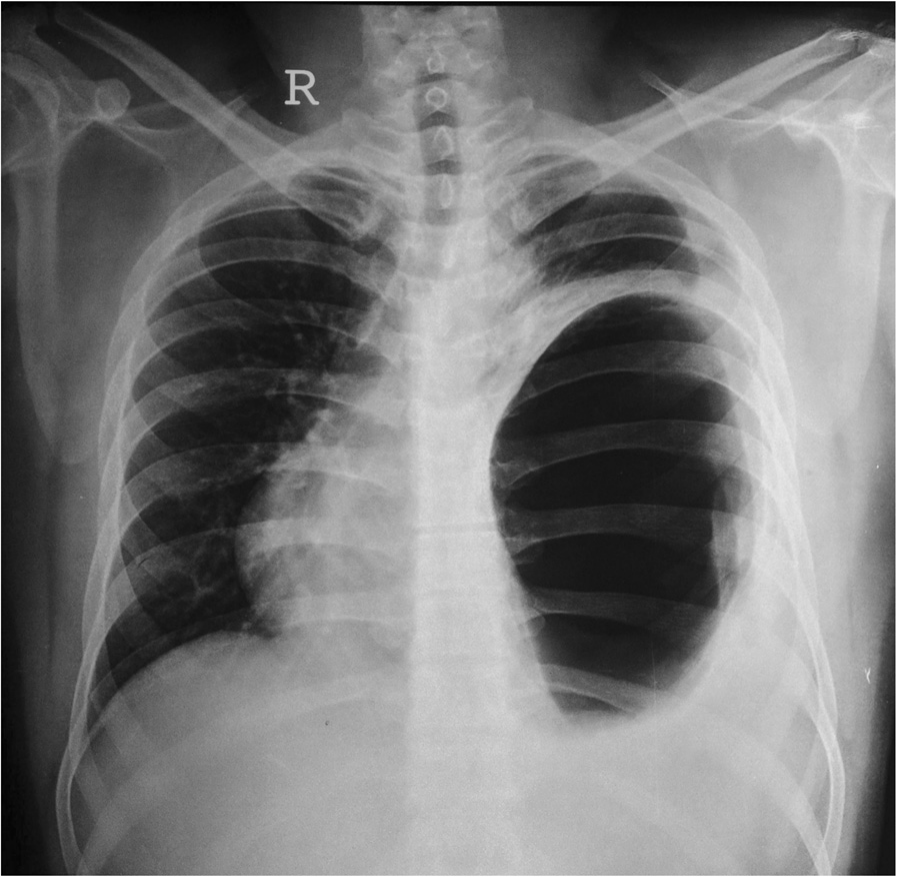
X-ray chest showing encysted hyper-translucence shadow covering the whole left hemi-thorax, with mediastinal shift to the right
